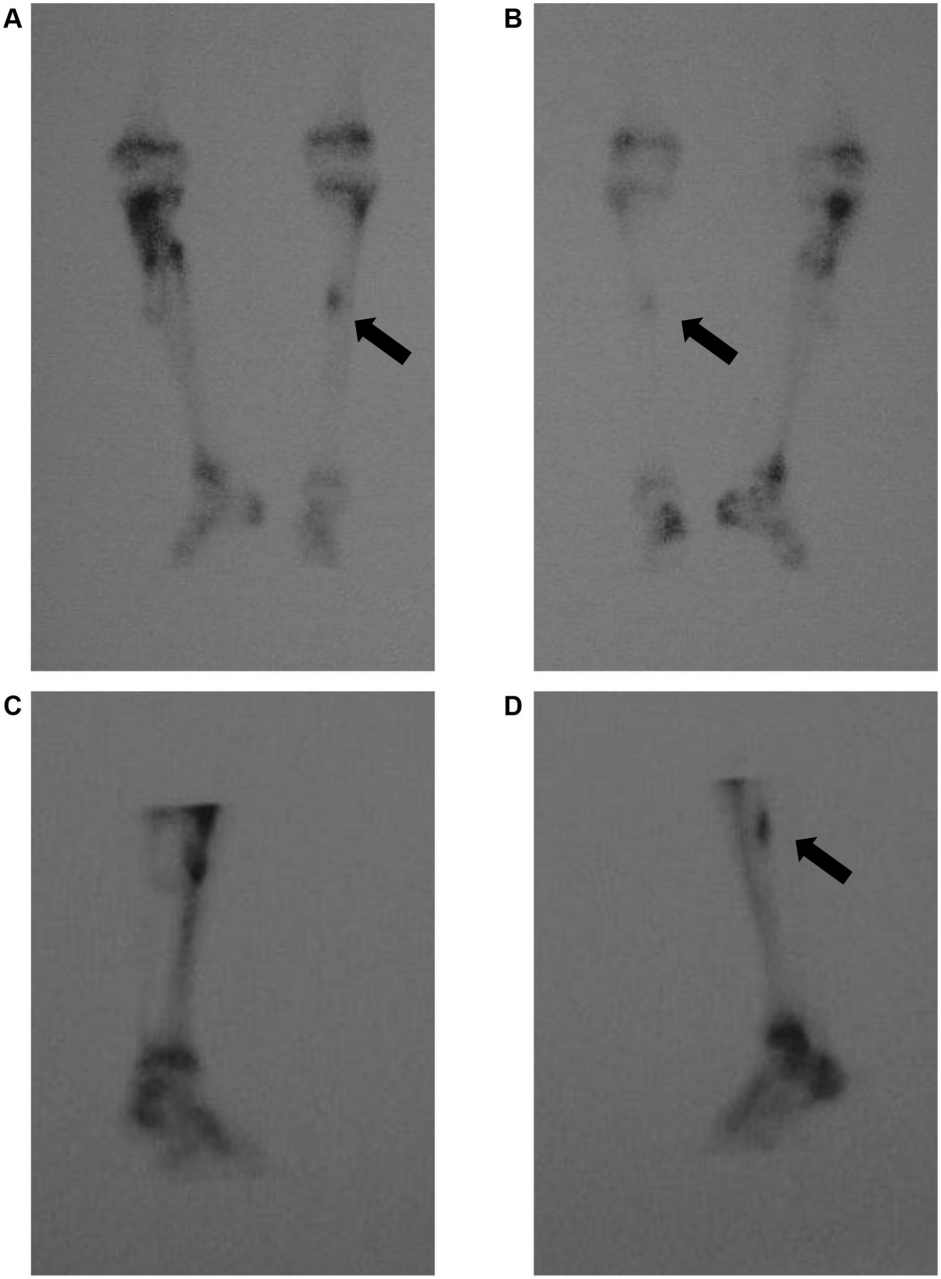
Bone scintigraphs show abnormal local uptake in the antero-posterior (A) and postero-anterior (B) and lateral views of the right side (C) and left side (D) of the patient with stress fractures. Arrows indicate longitudinal linear uptake in the bone scintigraph views of the patient with stress fractures
